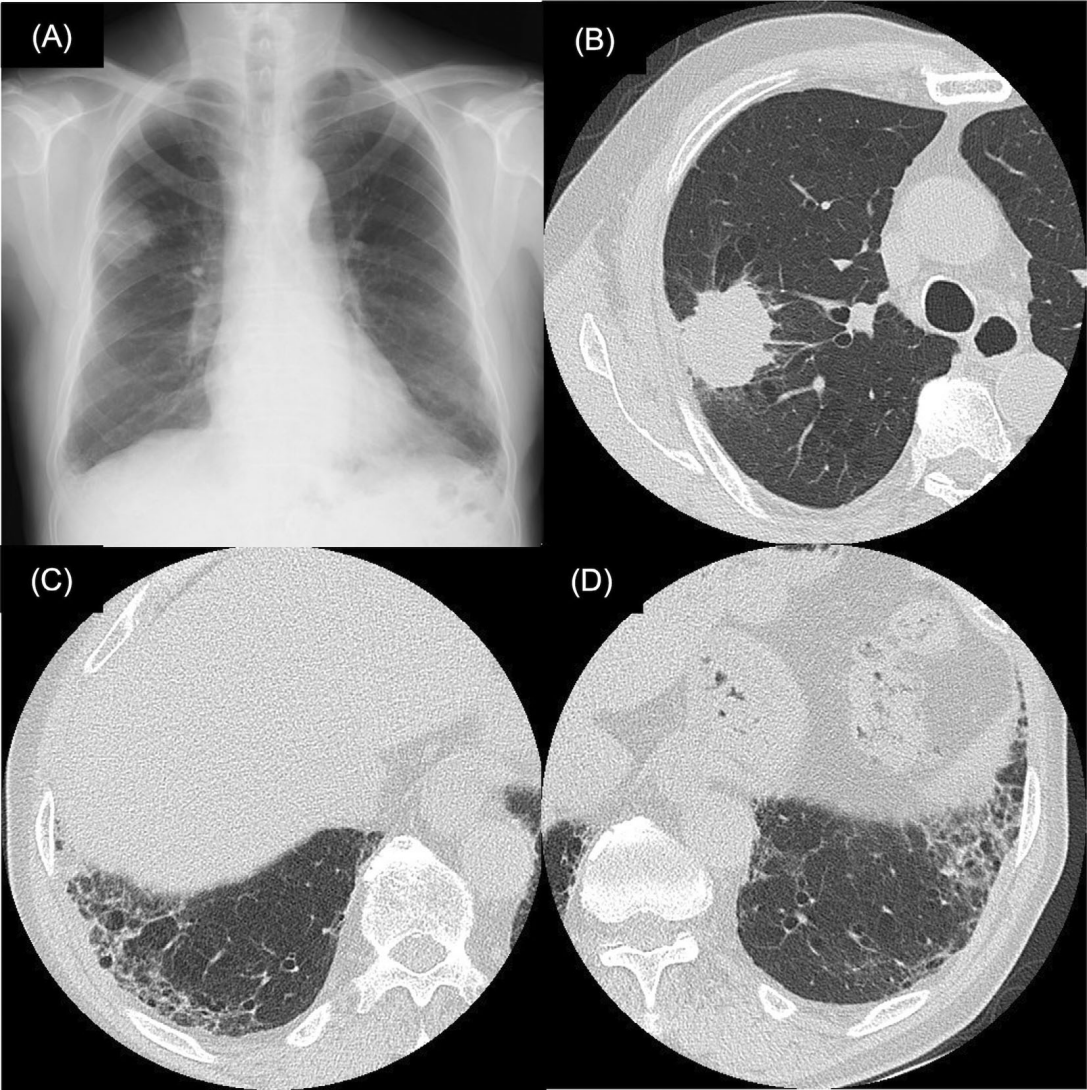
(A) Chest X‐ray findings at the time of referral to our hospital. High‐resolution computed tomography in the upper lung (B) and lower lung (C, D)
